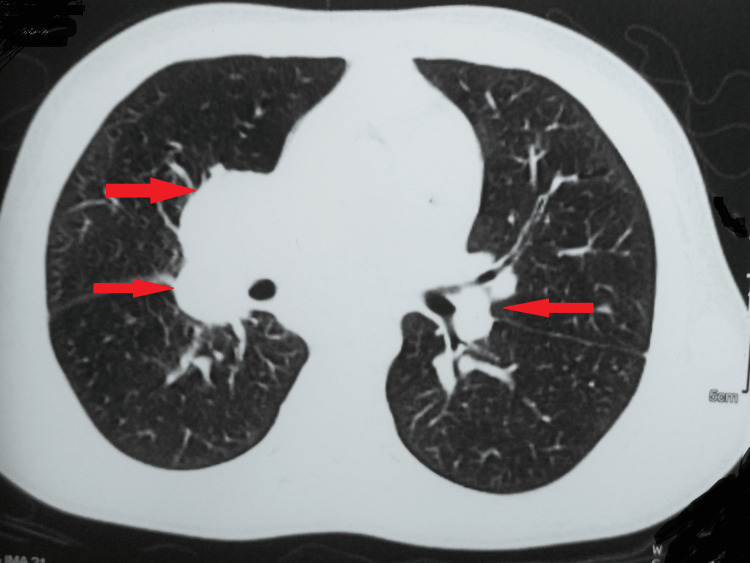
Computed tomography of the chest Arrows showing multiple enlarged conglomerated necrotic lymph nodes in the mediastinum
